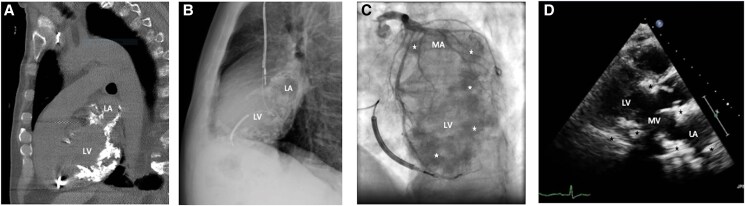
Multimodal cardiac imaging for the detection of heart calcifications. (*A*) Non-contrast computed tomography showing extensive amorphous confluent calcifications in the left ventricular wall, sparing the septum, and extending to the mitral-aortic annulus and the left atrium. (*B*) Chest radiography showing calcifications in the left ventricular wall, extending to the mitral-aortic annulus and the left atrium. (*C*) Calcifications (marked with stars) in the left ventricular myocardium and the mitral annulus as seen on fluoroscopy during the coronary angiography. (*D*) Transthoracic echocardiographic parasternal long-axis view showing the extensive calcifications (marked with black stars) of the mitral valve, extending to the mitral-aortic annulus, with intramyocardial calcification of the posterobasal and basal anteroseptal left ventricular walls, and significant calcification of the left atrium.
